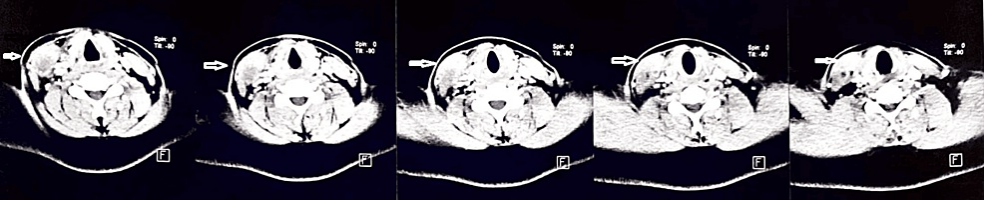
Axial cuts of contrast-enhanced CT images The white arrows show a hypodense lesion in the right sternocleidomastoid muscle CT: computed tomography
